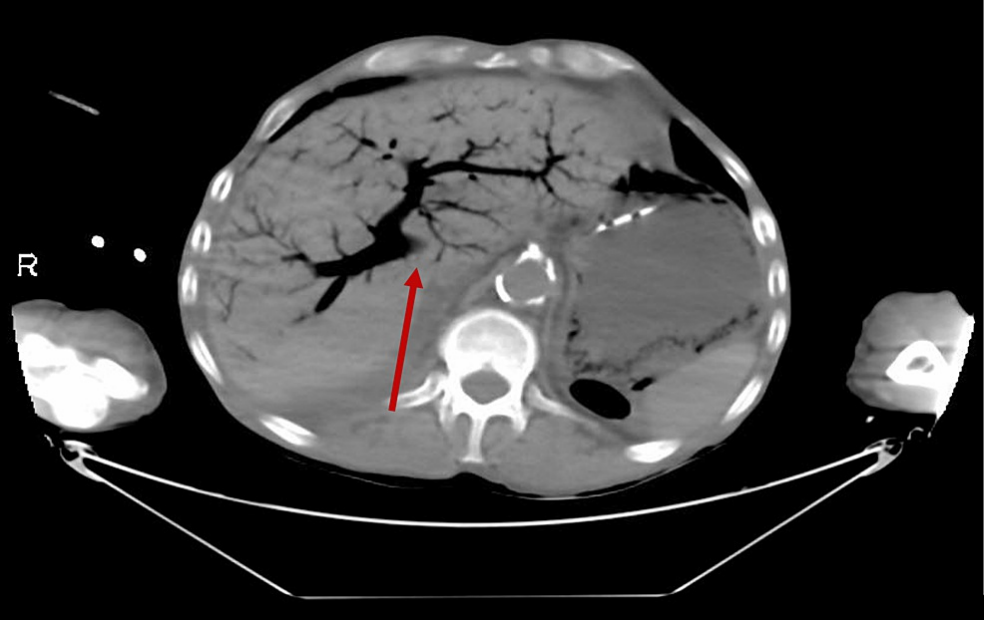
CT Abdomen/Pelvis (axial view) The image is showing HPVG (Hepatic Portal Venous Gas)
